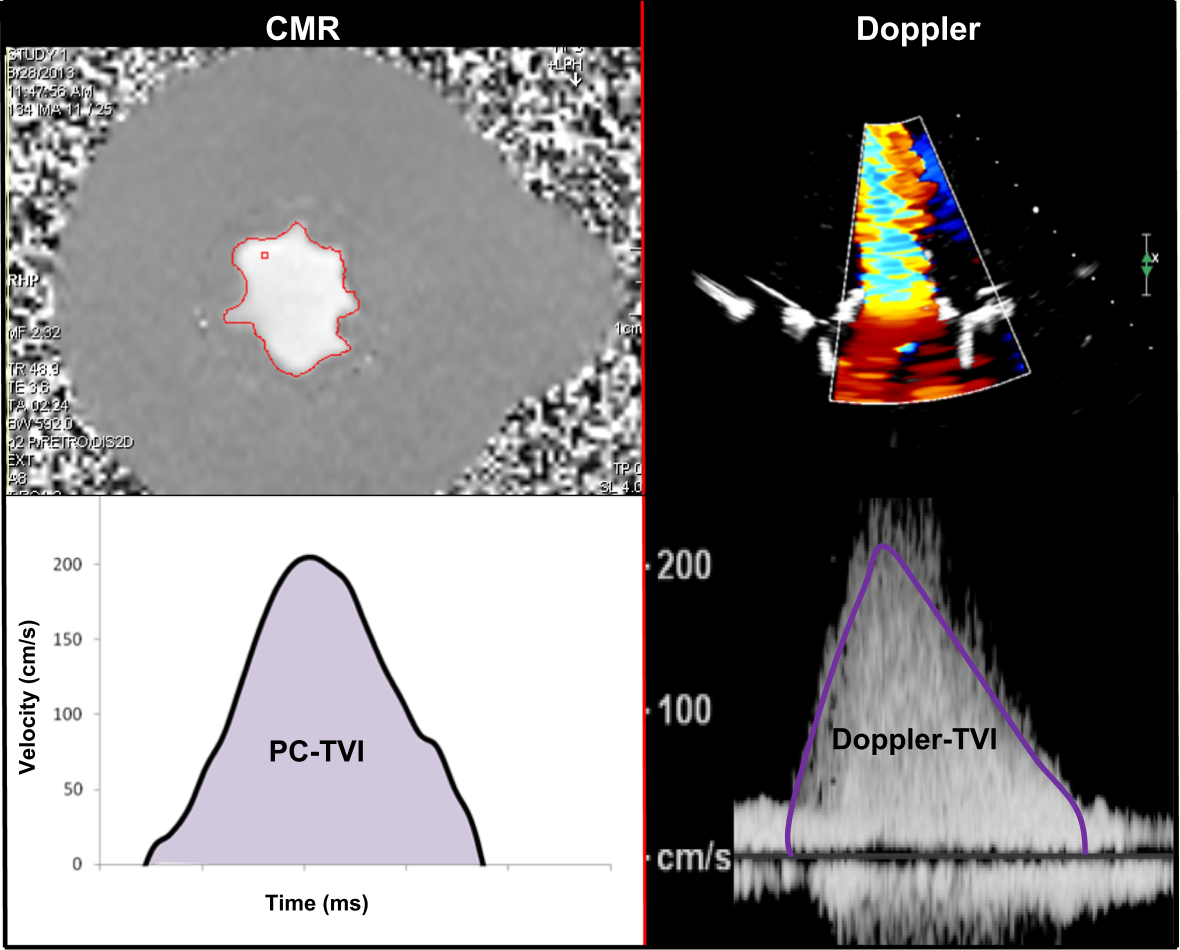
CMR and Doppler-derived time velocity integral assessment. Phase contrast image for a 31 mm BMV at a forward flow volume of 90 mL/beat. Phase contrast time velocity integral (TVI) by CMR (*left*), and spectral Doppler (*right*)
